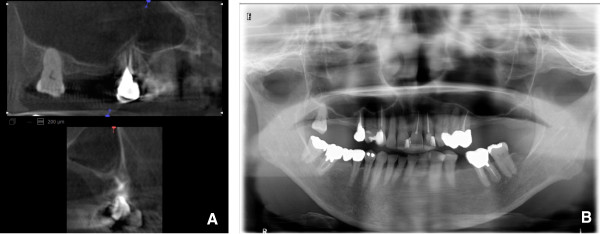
Images of pneumatization in the maxillary sinus. The CBCT (A) and panoramic radiograph (B) of a 68-year-old female undergoing preoperative planning for implantation of a left maxillary molar. The pneumatization is clearly visualized in the palatine area and anterior of the maxillary sinus on CBCT (A), but not on panoramic radiographs (B).
